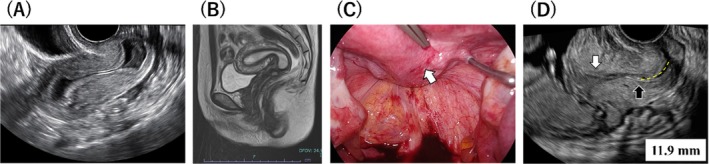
Imaging findings of hematometra, laparoscopic cervical recanalization, and cervical morphology at 9 weeks of gestation. (A) Transvaginal ultrasonography demonstrating blood accumulation within the uterine cavity and cervical canal. (B) Sagittal T2‐weighted magnetic resonance image demonstrating marked distension of the uterine cavity and cervical canal caused by severe cervical stenosis. (C) Laparoscopy‐assisted recanalization. The original cervical canal was difficult to identify despite laparoscopic guidance, and multiple false passages were created during the initial recanalization attempts. The uterine cavity was ultimately accessed using a central venous catheterization kit, followed by cervical reconstruction and dilation. The white arrow indicates the actual catheter placement site. (D) Transvaginal ultrasonography at 9 weeks of gestation showing a residual endocervical glandular region measuring 11.9 mm from the external cervical os to its cranial end (black arrow). The white arrow indicates the anatomical internal cervical os.
